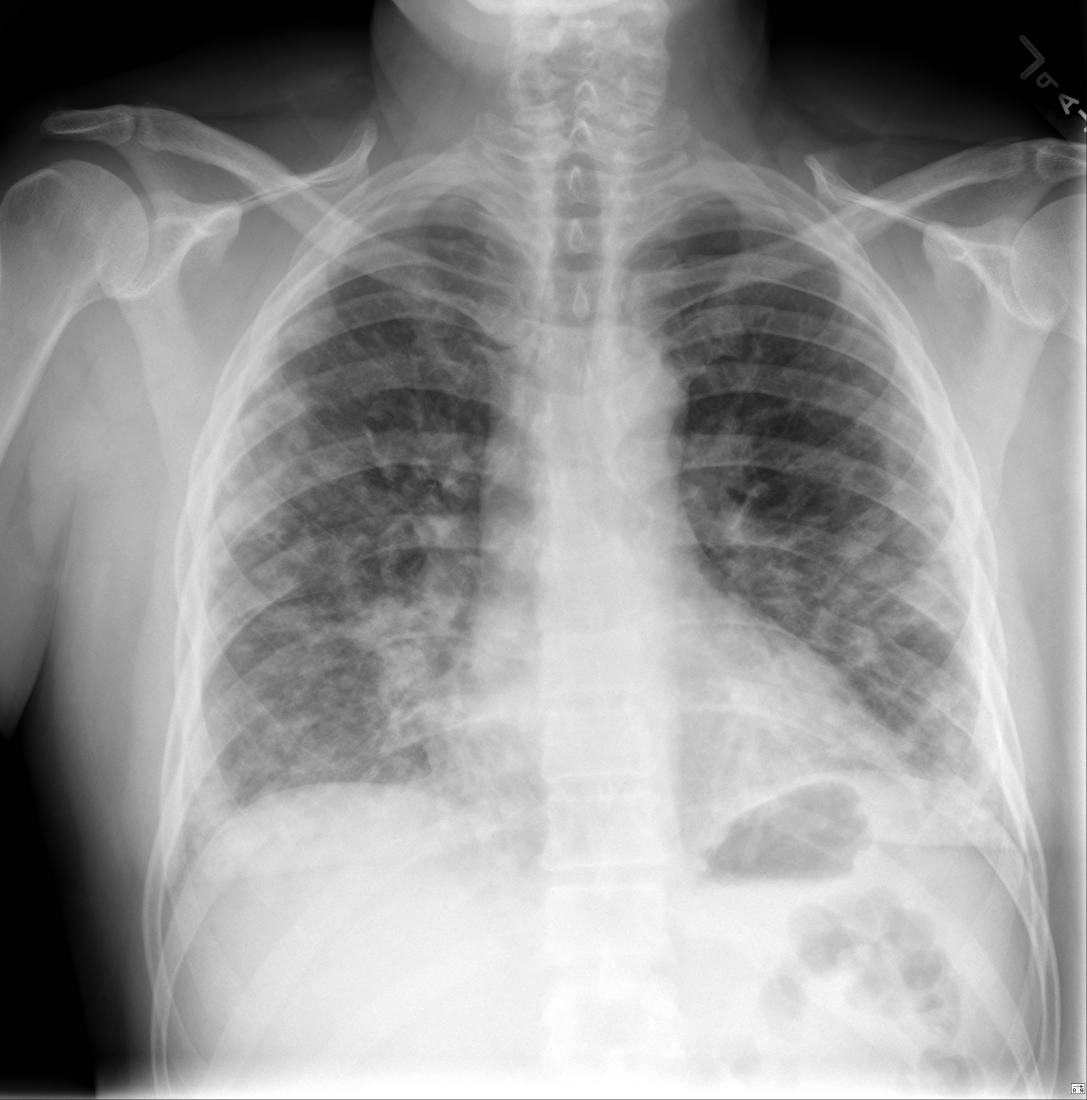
Chest X-ray (PA view): adjacent left basilar infiltrates and mild atelectasis. No infiltrates are seen on the right side
